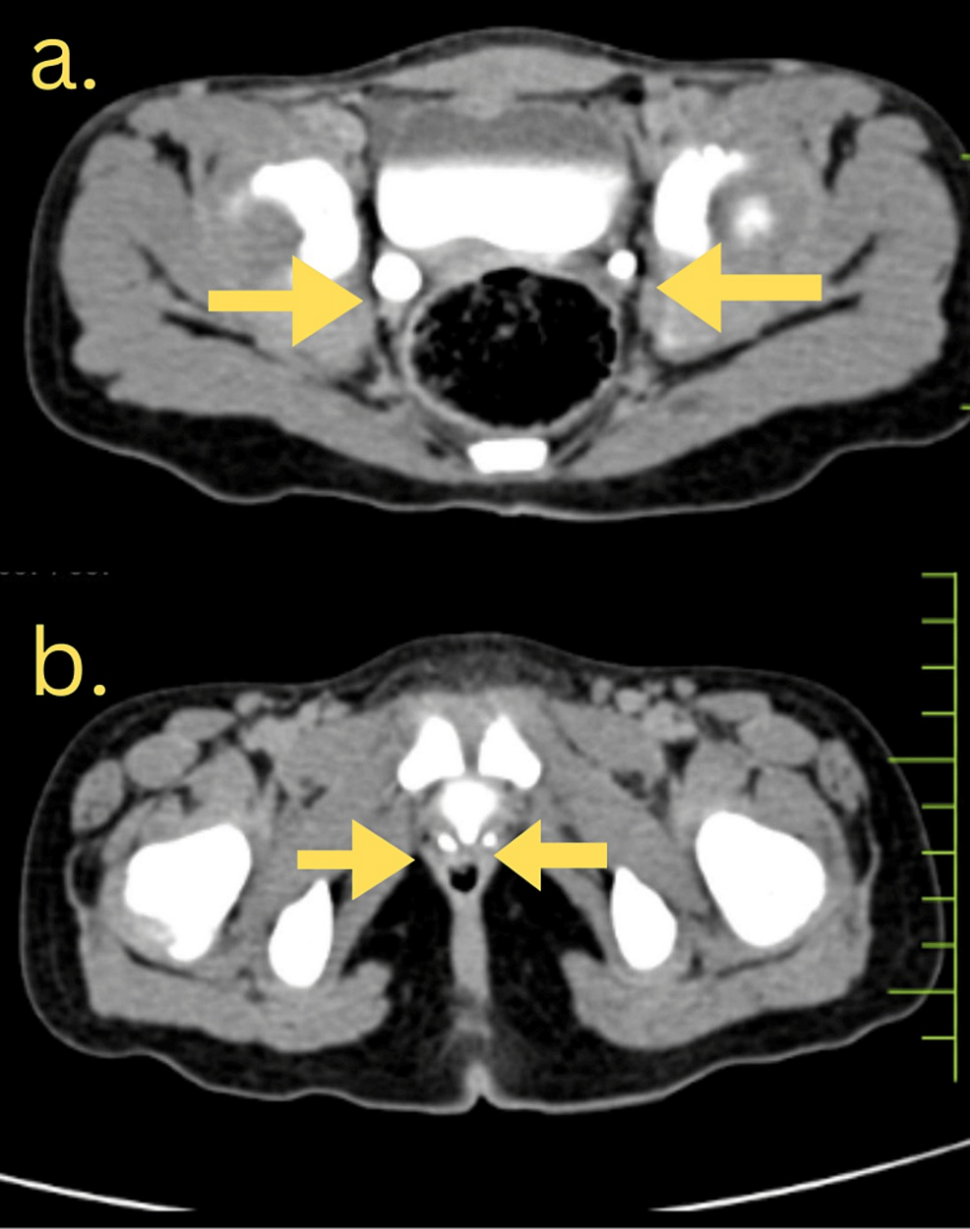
CT urography dilated bilateral ectopic ureters
